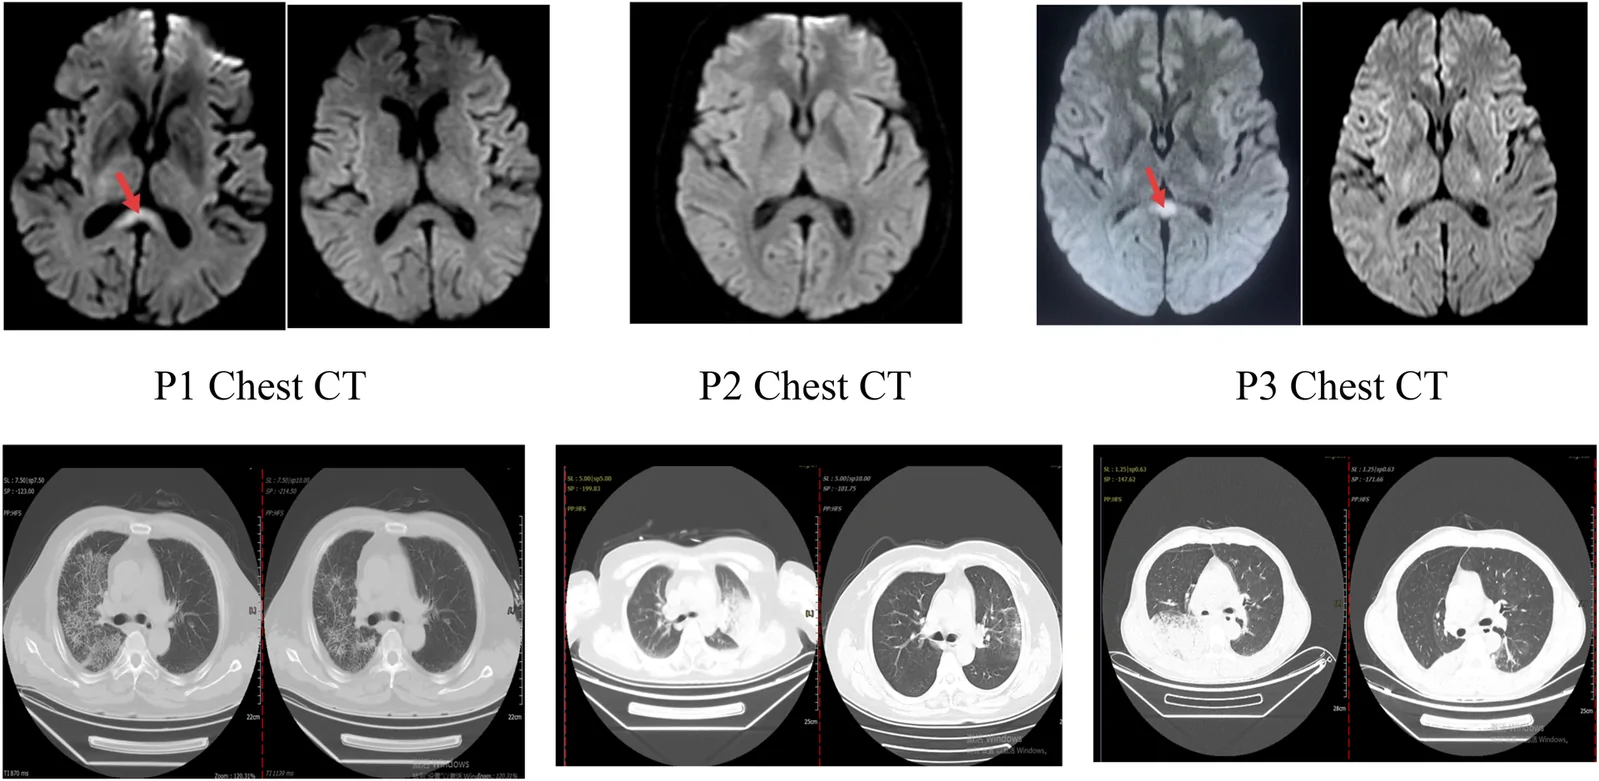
Available brain MRI and paired baseline/follow-up chest CT images. Brain MRI is shown at baseline and follow-up for P1 and P3 and at baseline only for P2. Arrows indicate the splenial DWI abnormalities in P1 and P3. All displayed images are de-identified.
